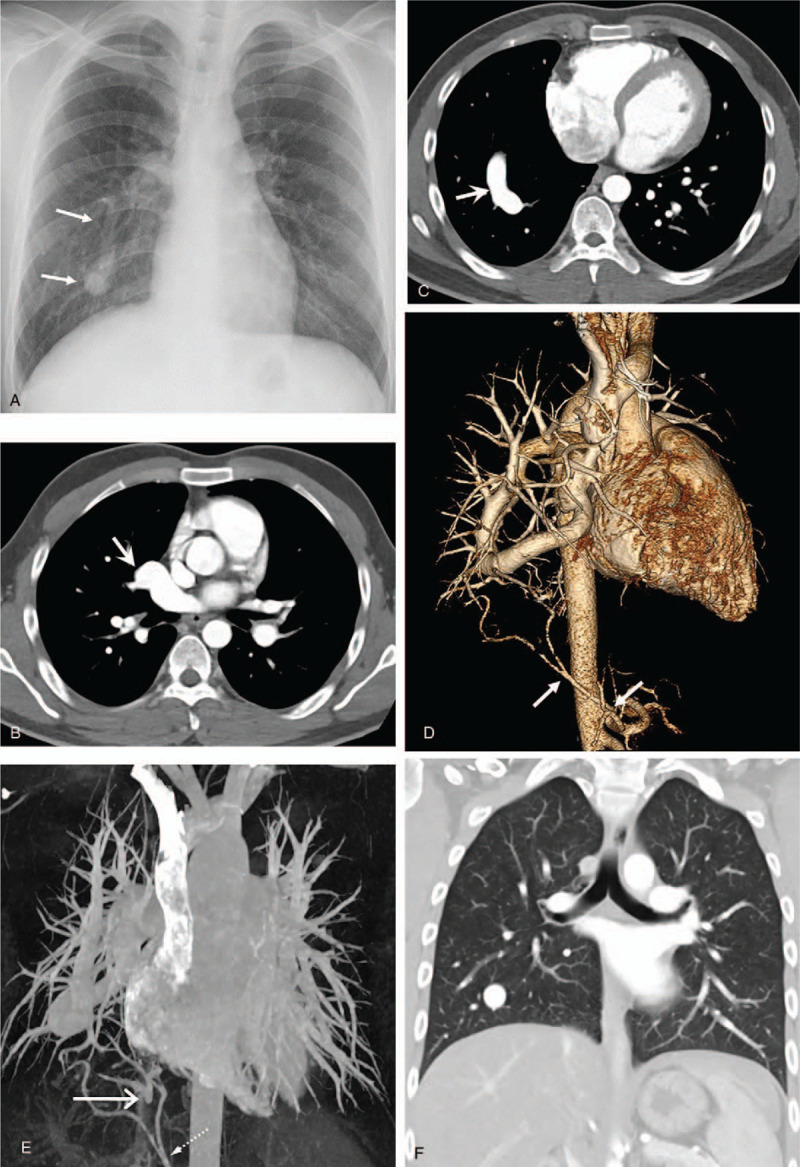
A 20-year-old man transferred for further evaluation of abnormal finding on chest radiograph. A. Chest radiograph demonstrating an elongated curvilinear structure (arrows) coursing vertically down the right heart border. B and C. Contrast enhanced chest CT images revealing an engorged anomalous pulmonary vein (arrow in B) emptying into the left atrium via left superior pulmonary venous ostium (arrow in C). There was no right inferior pulmonary vein (not shown here). D. Three-dimensional volume rendered image showing focal systemic arterial blood supply from the abdominal aorta in the posterior basal segment of the right lower lobe (arrows). E. Maximum intensity projection image demonstrating small pulmonary venous branches emptying into IVC (long arrow). Also note systemic arterial supply from abdominal aorta (dashed arrow). F. Coronal reformatted image with lung window setting showing 2 bilobed lungs with bilateral hyparterial bronchial branching pattern (left bronchial isomerism).
